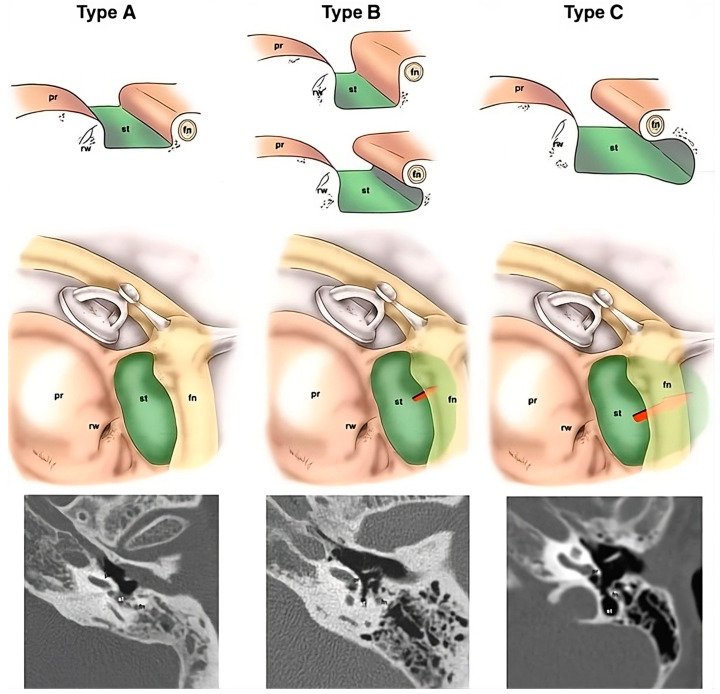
Classification of depth of the sinus tympani, based on an axial CT scan. Type A: small sinus tympani. Type B: deep sinus tympani. Type C: deep sinus tympani with posterior extension. Fn, facial nerve; pr, promontory; rw, round window; st, sinus tympani.
